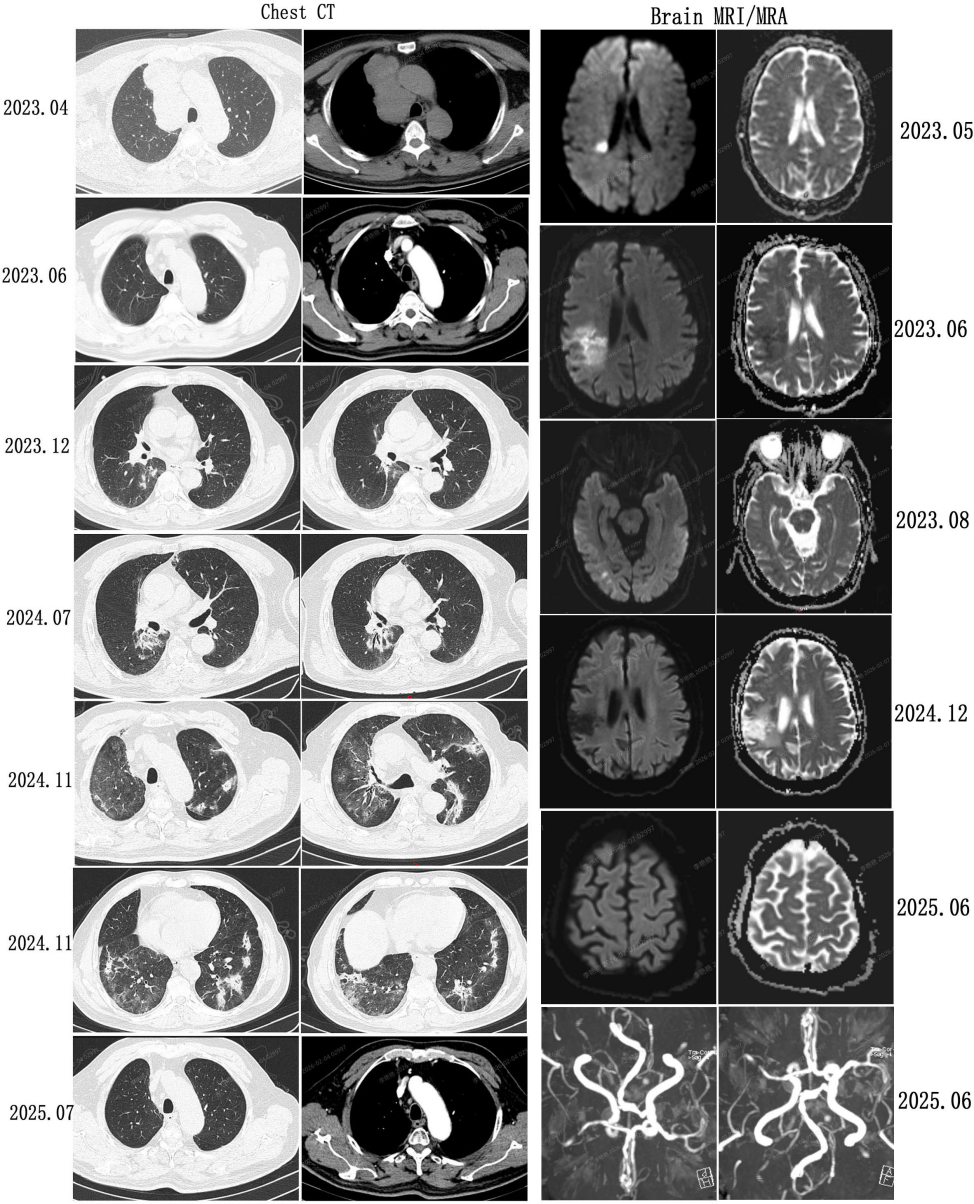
Representative serial images of chest CT, brain MRI, and brain MRA. Brain MRI sequences: diffusion-weighted imaging (DWI, left) shows a hyperintense lesion, and the corresponding apparent diffusion coefficient (ADC) map (right) demonstrates hypointensity, consistent with a subacute cerebral infarction. MRA reveals severe stenosis of the right middle cerebral artery.
